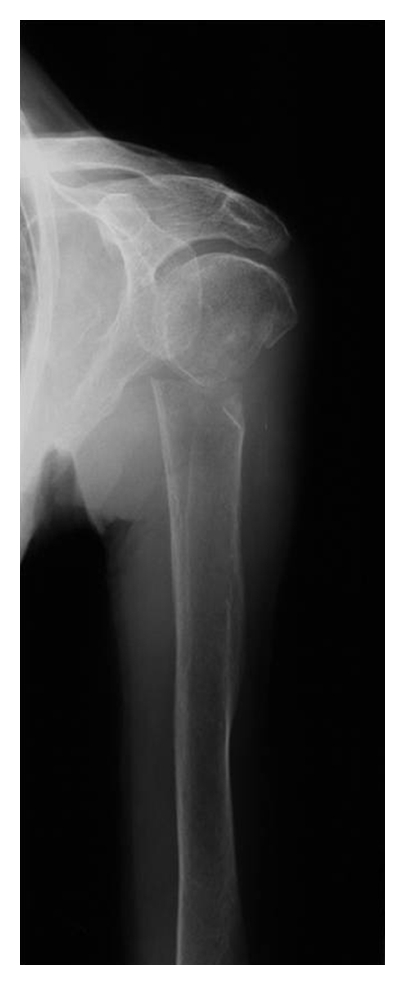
Initial X-rays of the dislocated proximal humeral fracture (AP-view).
